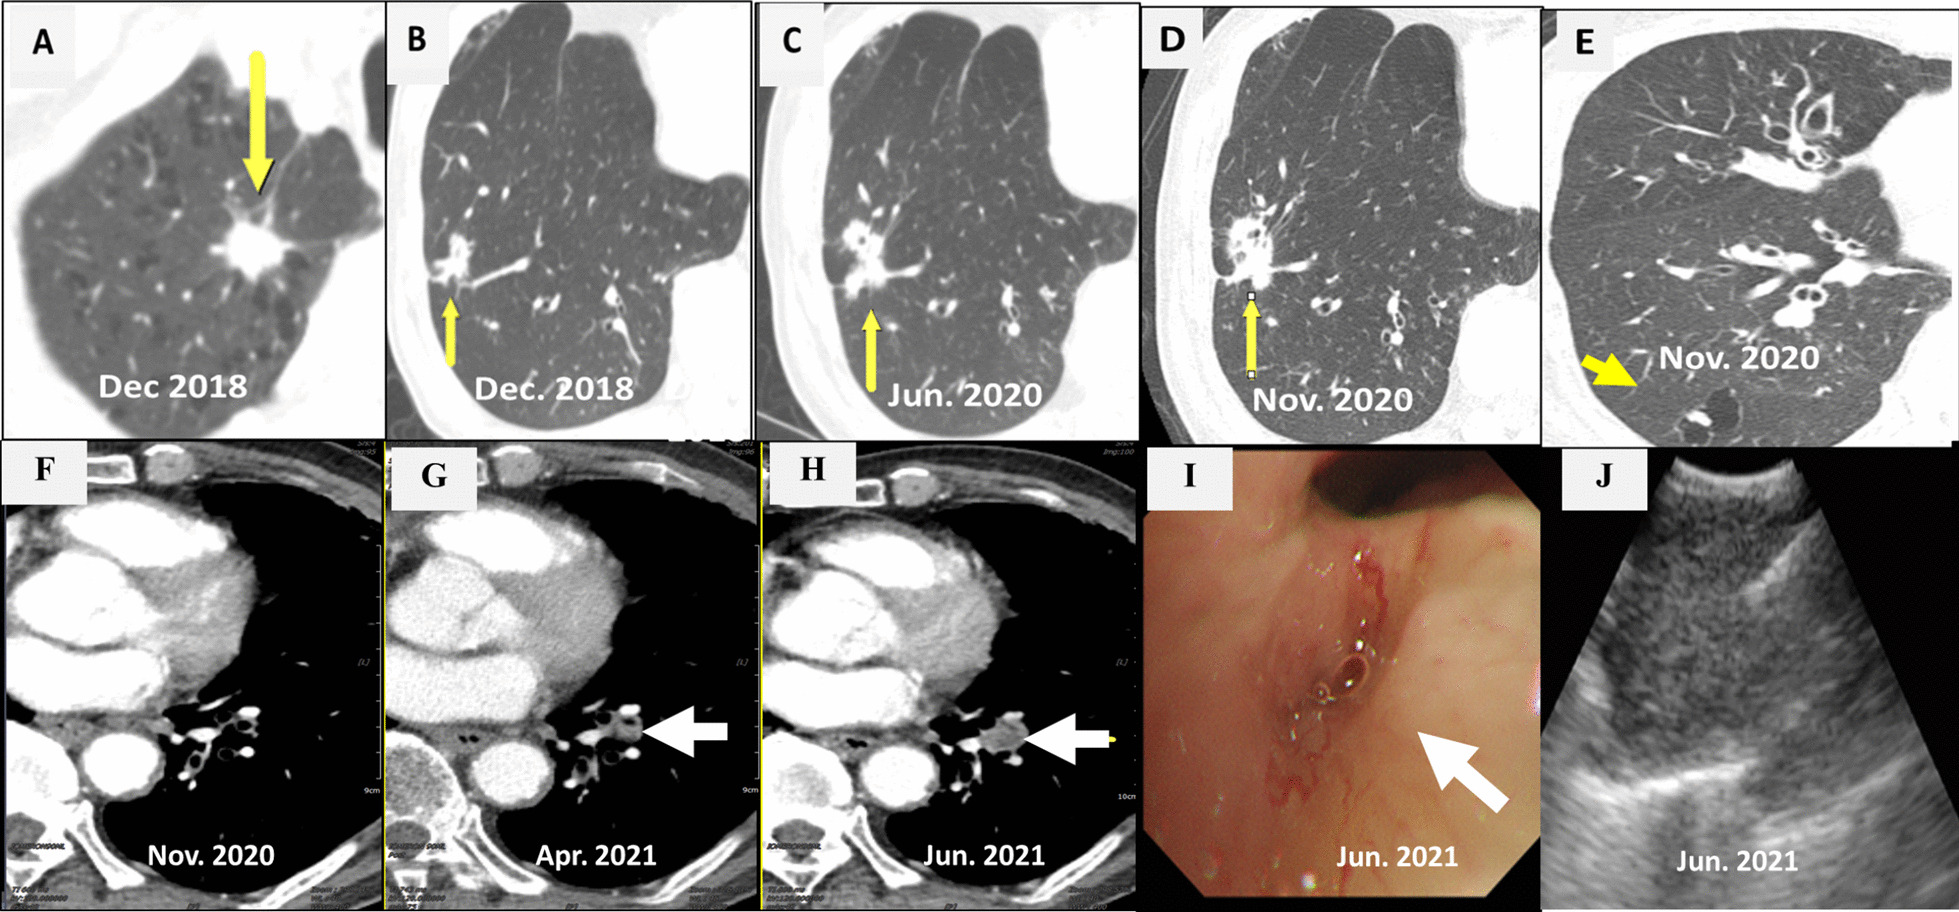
Chest CT scans. a A mass on the RUL of the first adenocarcinoma (arrow). b A mass on the RLL at the same time of the first cancer diagnosis (arrow). c Increased RLL mass six months later (arrow). d Further increased RLL mass after five months (arrow). e New nodule on the peripheral RLL (arrow). f–h Development and increase of the lymph node (arrow). i Bronchoscopic finding showing LLL anterobasal segment obstruction (arrow). j Lymph node enlargement on the EBUS. CT, computed tomography; RUL, right upper lobe; RLL, right lower lobe; LLL, left lower lobe; EBUS, endobronchial ultrasound
